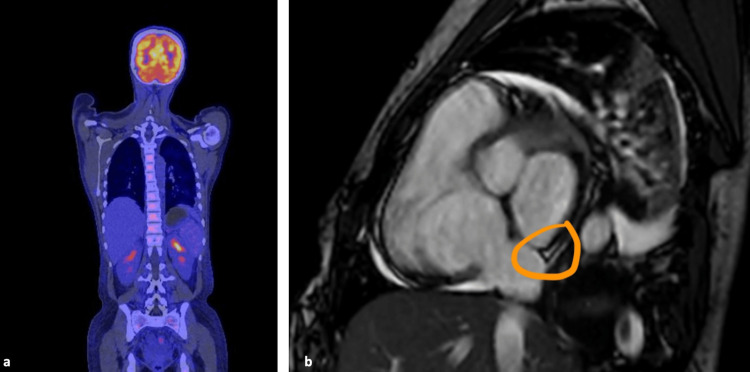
Positron emission tomography CT scan (PET-CT) images a) Bilateral retroperitoneal and pelvic adenopathies with mild increase in metabolism; b) Late linear enhancement in the subepicardial band in the basal and mesial inferolateral segments and lateral apical segment. Myocardium with enhancement =1% of the end-diastolic mass.
